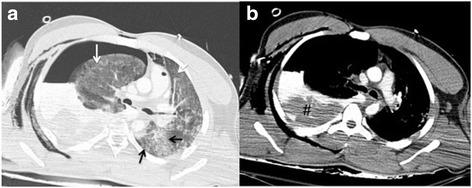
CT-images of the chest after falling from a great height demonstrate in the lung window (a) extensive lung parenchymal lacerations (see *black arrows*) along with contusions of the lung (see *white arrows*). Pleural effusion (soft tissue window, b demonstrates blood-like density values along with condensed portions in terms of hematothorax (see #) and corresponding mediastinal shift towards the left side. However, the exact source of bleeding was only suspected and evaluated as bleed to death but not exactly detected on pmCT but stated by the gold standard autopsy in terms of aortic rupture as cause of death
